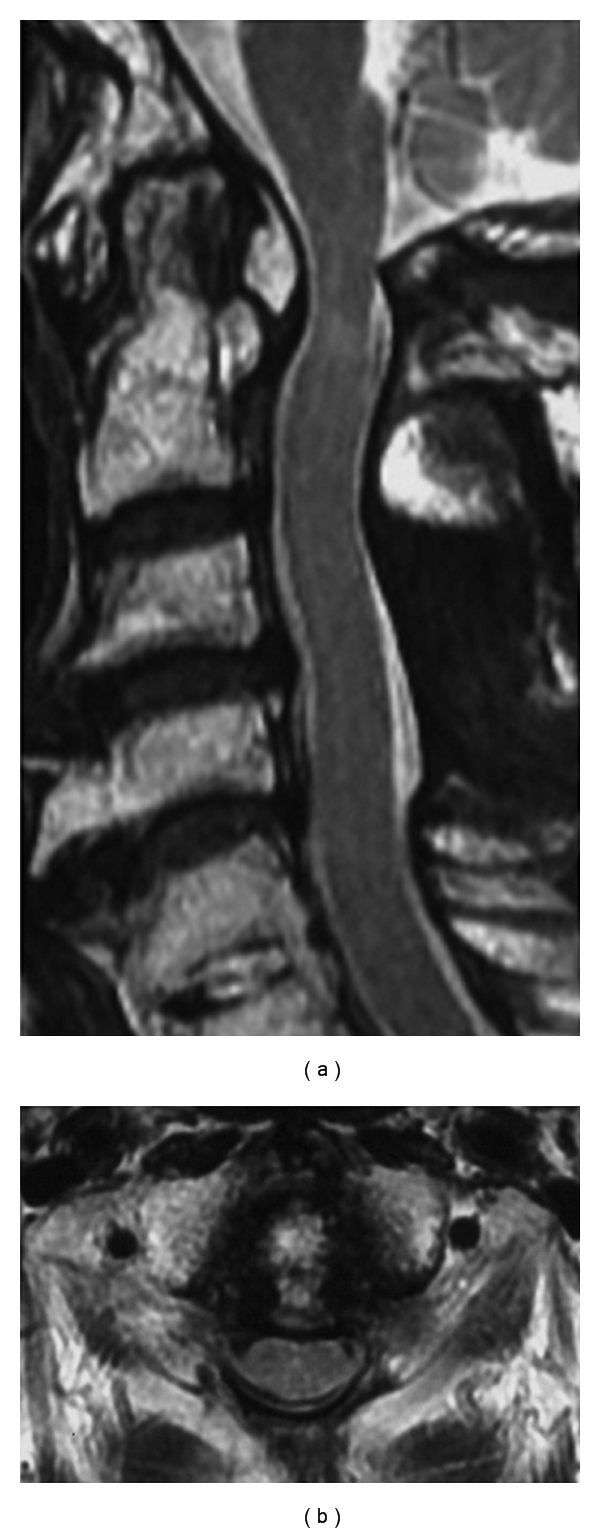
Postoperative MRI on T2-weighted image. Sagittal (a) and axial (b) plane. The spinal cord had been decompressed.
